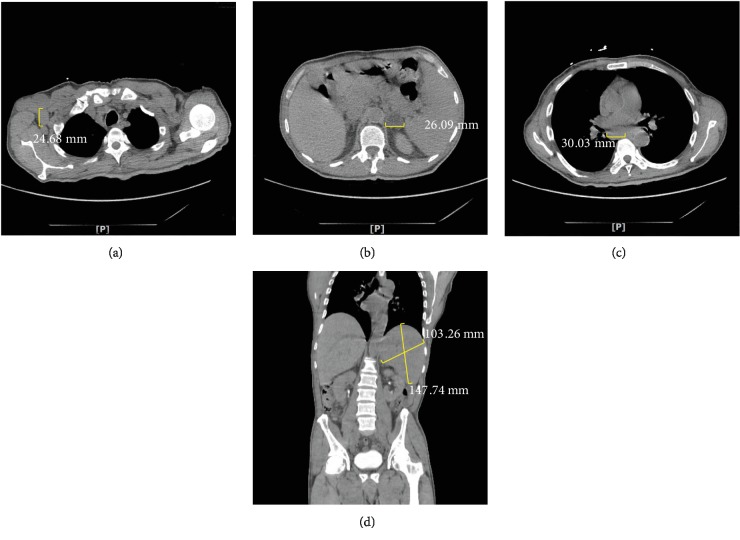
CT of the chest, abdomen, and pelvis. (a) Axillary lymphadenopathy. (b) Retroperitoneal lymphadenopathy. (c) Paraaortic lymphadenopathy. (d) Splenomegaly.
